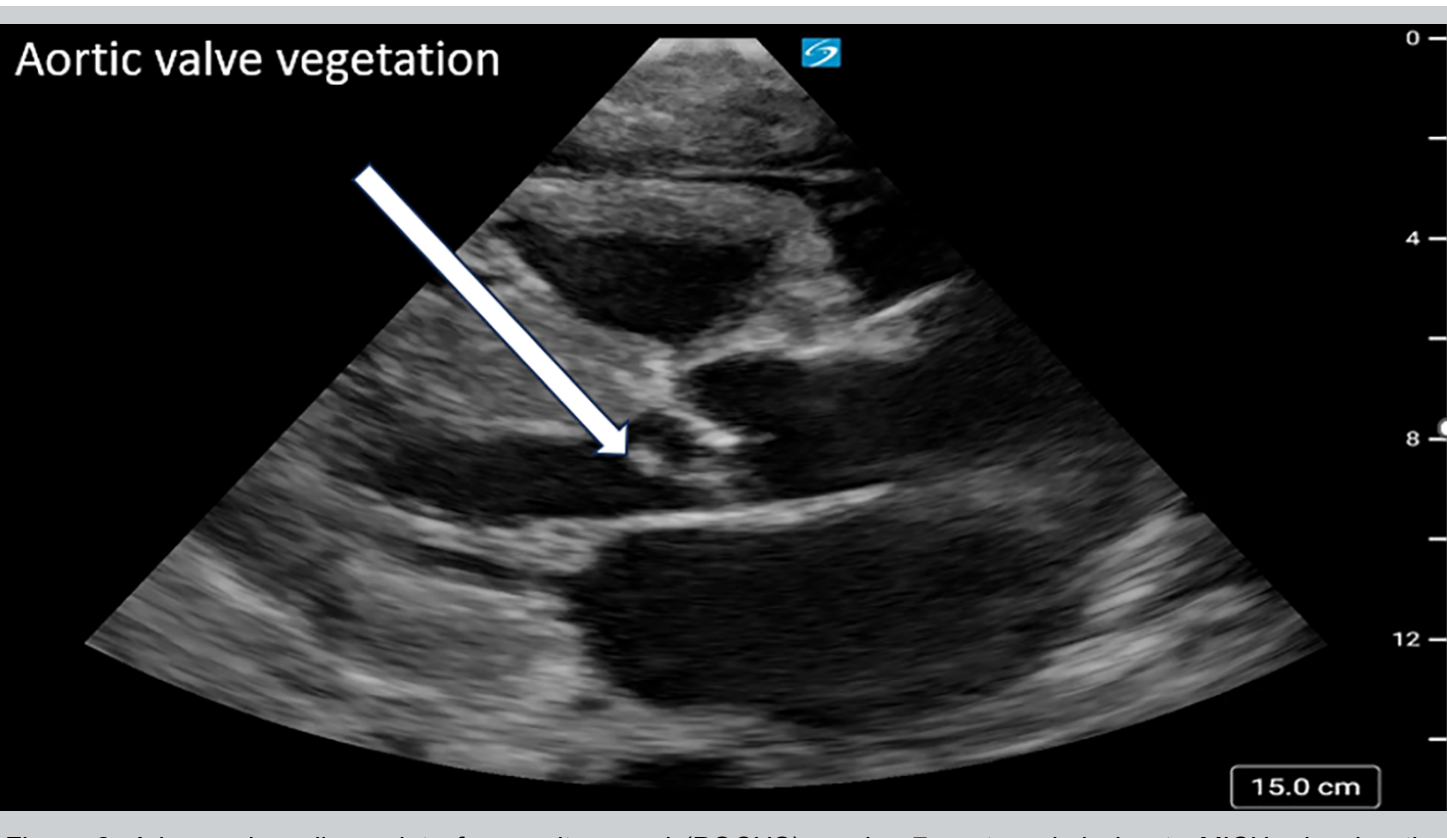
Advanced cardiac point of care ultrasound (POCUS) on day 7 post-readmission to MICU, showing the parasternal long axis view demonstrating an aortic valve vegetation.
